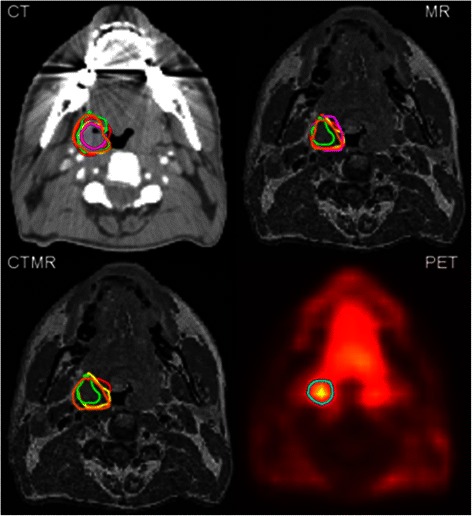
Example of inter-observer variability in contouring GTVs based on CT, MR, CT-MR and of auto-segmented contour based on PET for a patient with a T2 N2b poorly differentiated squamous cell carcinoma of the right tonsil. Contours shown are: radiation oncologist 1 red, radiation oncologist 2 yellow, radiation oncologist 3 orange, radiologist 1 green, radiologist 2 purple, PET contour blue
